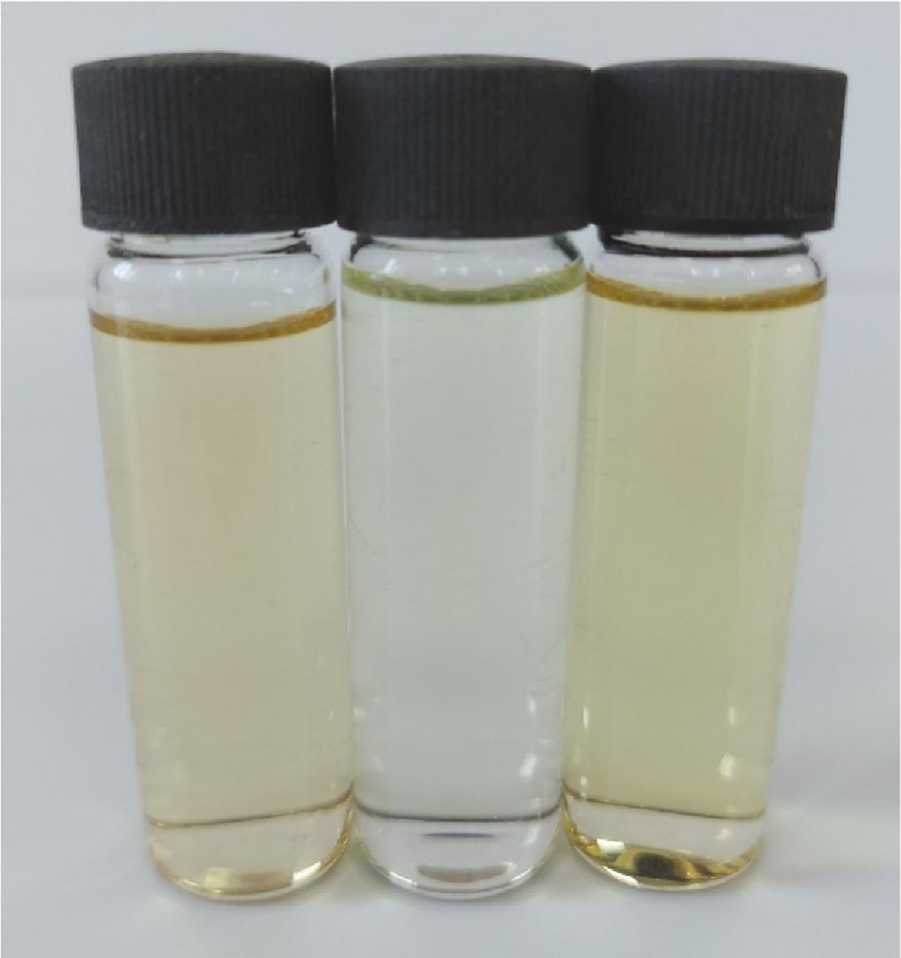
Color change upon mixing TFQ acceptor (*far left; pale brown*) with the donor (*middle; colorless*) to generate the CT complex (*far right; light brown*). (For interpretation of the references to color in this figure legend, the reader is referred to the web version of this article.)
